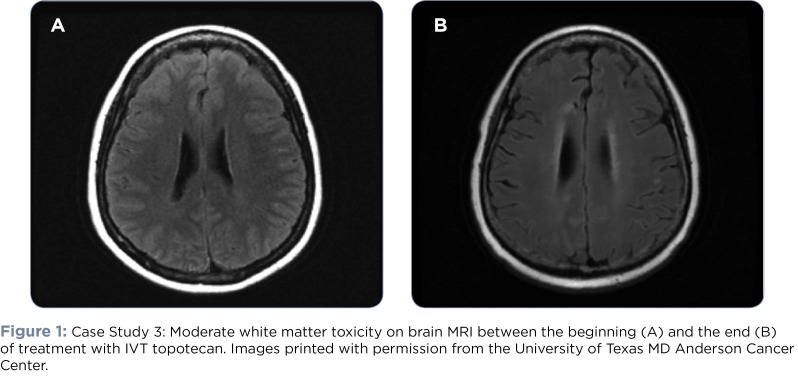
Figure 1: Case Study 3: Moderate white matter toxicity on brain MRI between the beginning (A) and the end (B)of treatment with IVT topotecan. Images printed with permission from the University of Texas MD Anderson Cancer Center.
